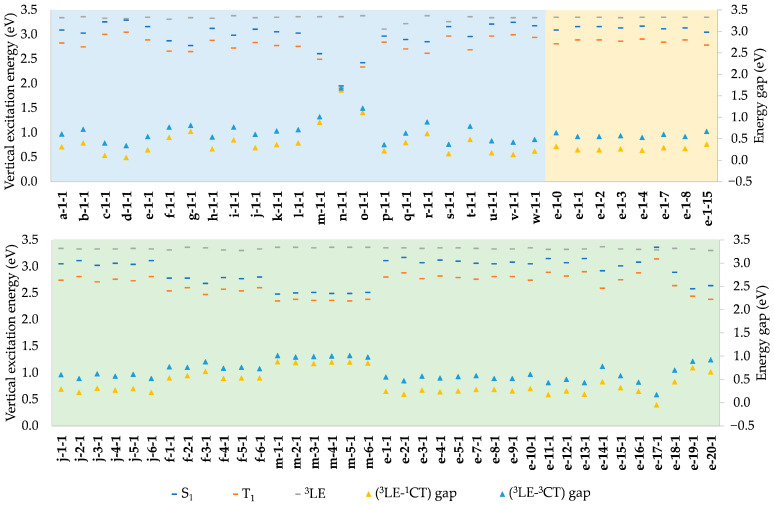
Vertical excitation energies and (^3^LE-^1^CT) and (^3^LE-^3^CT) gaps of complexes modified at positions 1 (blue), 2 (green), and 3 (yellow), where ^1^CT and ^3^CT are singlet and triplet CT characters, respectively.
